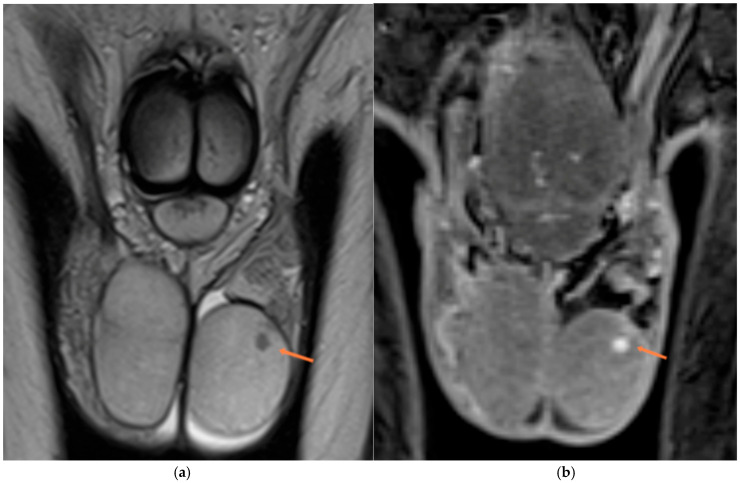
Leydig cell tumor (orange arrows). (a) Coronal T2-weighted image shows a small, well-defined hypointense intratesticular lesion. (b) Corresponding contrast-enhanced T1-weighted image demonstrates intense, homogeneous enhancement of the lesion.
